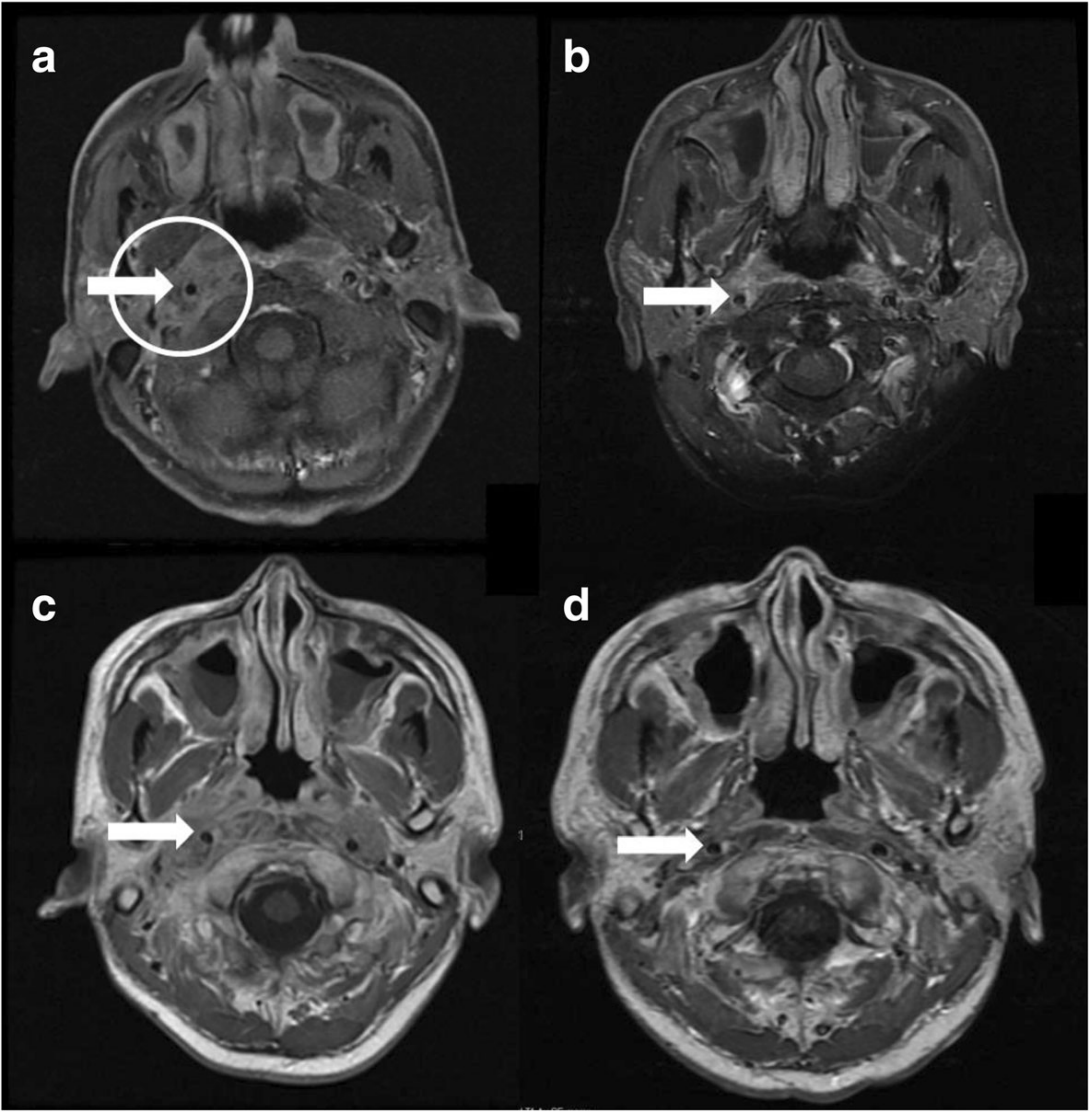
Magnetic Resonance (MR) imaging of the head. Fat-suppressed T1-weighted gadolinium-enhanced axial scans through the skull base (a, b), and T1-weighted gadolinium-enhanced axial scans of the skull base (c, d). There is bilateral maxillary sinusitis and an infiltrative lesion of the right retropharynx (circle) around the internal carotid artery (arrow). Images were taken a on admission, b after the 1st IVCY, c before RTX treatment, and (d) 6 months after RTX treatment. (d) The sinusitis and the infiltrative lesion were markedly improved after RTX treatment
